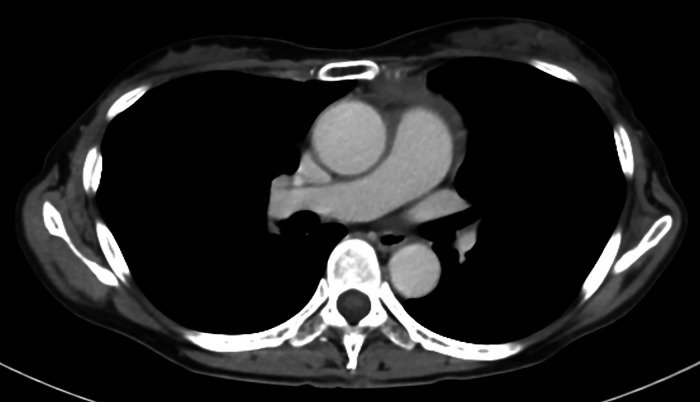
Axial images of contrast-enhanced CT-scan at 3 months after starting treatment in the first case. Axial CT scan image showing shrinkage of the mediastinal mass.
